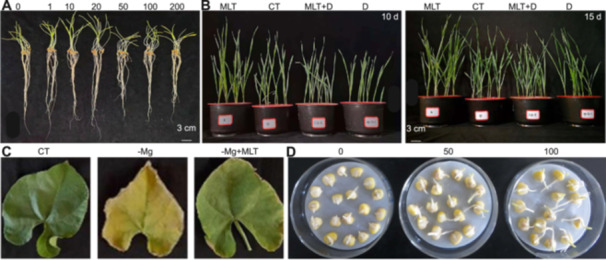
Phenotypic traits of different plants harvested with melatonin (MLT) seed priming (A) Germination of MLT (μM)‐primed triticale wheat seeds after 7 d. (B) Phenotype of triticale seedling in pot cultivation under drought stress and with MLT treatment. (C) Common bean leaves cultivated in hydroponics with a magnesium (Mg)‐sufficient solution (CT), a Mg‐deficient solution (−Mg), and a Mg‐deficient solution with MLT‐primed seeds (−Mg + MLT). (D) Morphology characteristics of maize seeds after 9 d of germination with various MLT concentrations (μM) for 24 h. These images were adapted and text‐edited from Open Access publications under Creative Commons Attribution License (CC BY International License; https://creativecommons.org/licenses/by/4.0/). Panels A and B were taken from (Guo et al., 2022); panel C was taken from (Bouzidi and Krouma, 2025); and panel D was taken from (Cao et al., 2019).
